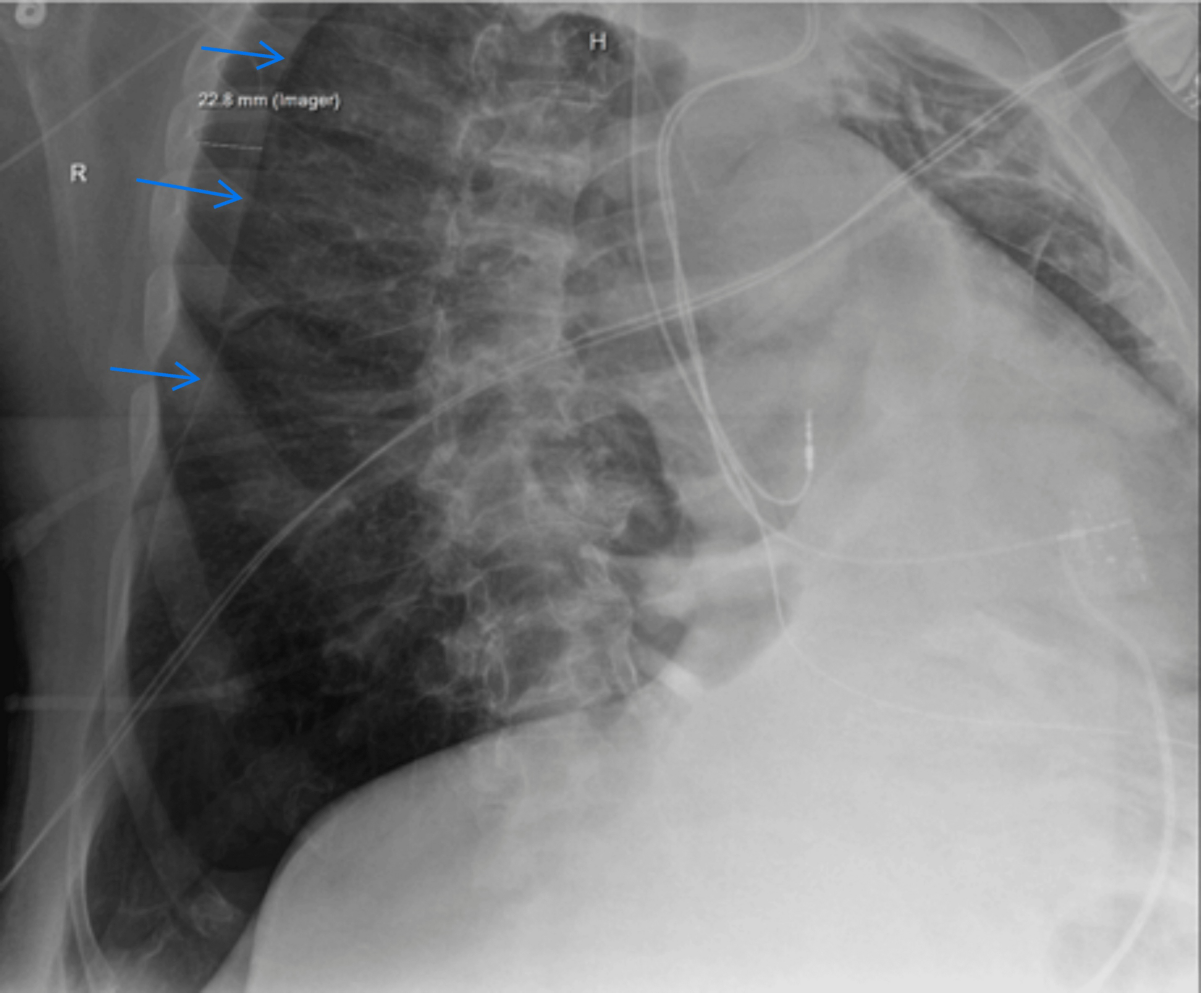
Intraoperative chest X-ray #1 Arrows show right-sided pneumothorax.
